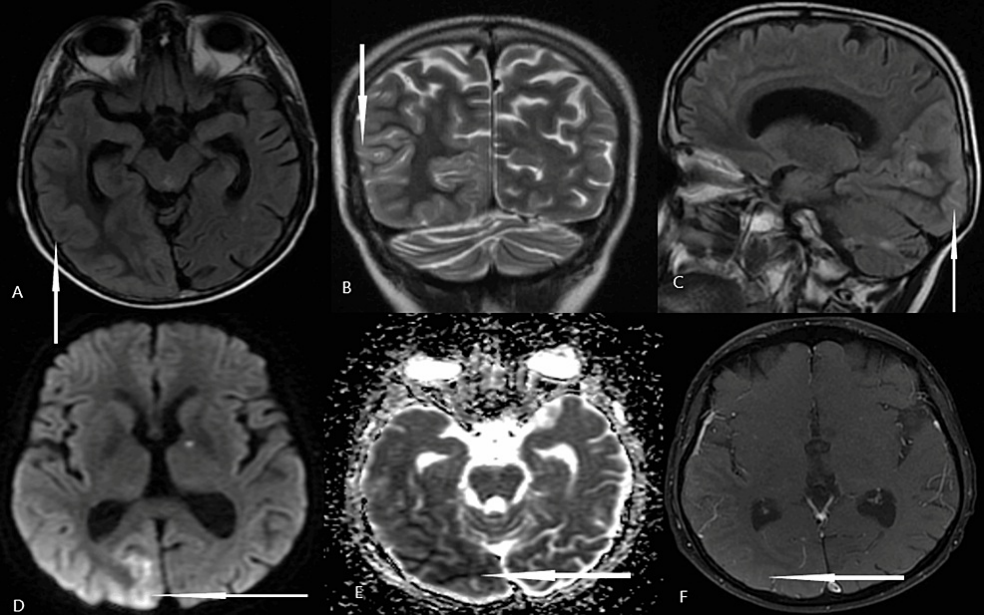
MRI brain images MRI brain: top panel (A) T2 FLAIR axial, (B) T2-weighted coronal (C) T2 FLAIR sagittal, showing cortical gyral edema involving right parieto-occipital area Bottom panel: (D) diffusion-weighted sequence showing diffusion restriction in the corresponding area, (E) apparent diffusion coefficient (ADC) sequence showing a drop in ADC value, and (F) T1 post-gadolinium sequence showing faint enhancement
